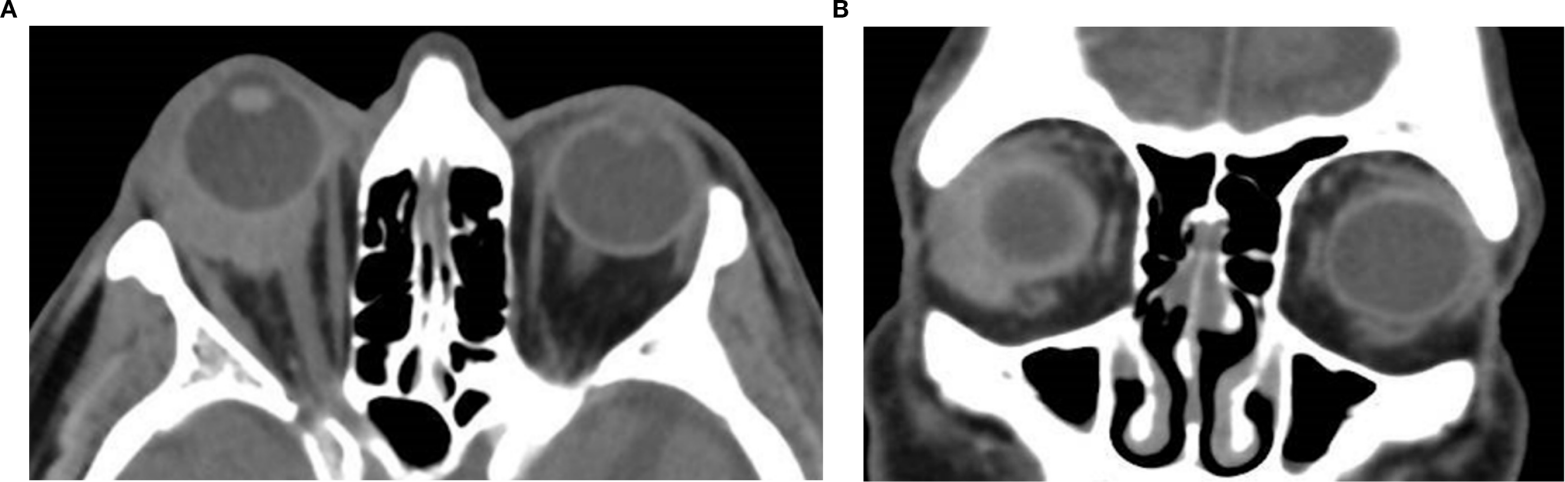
Axial (a) and coronal (b) non-contrast CT image of a 74-year-old male patient showing a homogeneous, well-defined soft tissue mass in the right lacrimal gland fossa. The lesion is hyperdense relative to orbital fat, demonstrates molding to adjacent structures with associated proptosis, and lacks bone erosion; biopsy confirmed it as lacrimal gland lymphoma.
